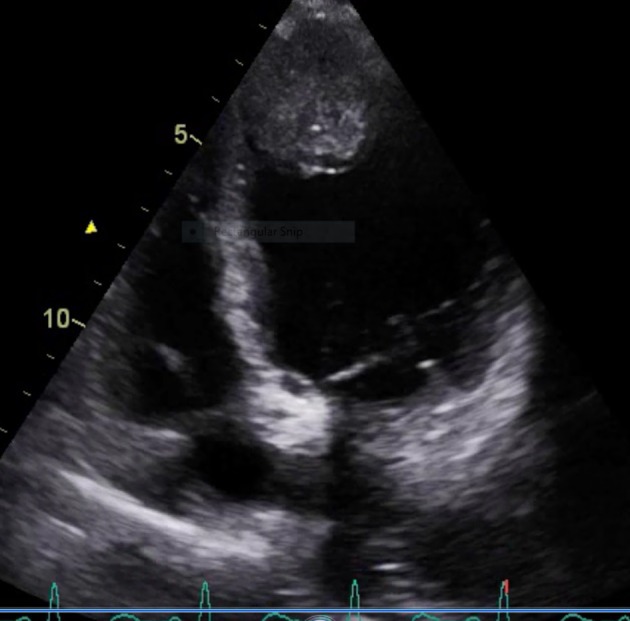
Apical two-chamber view showing heterogenous echogenic irregular vascular mass lesion in the endocardium with normal apical mobility.
